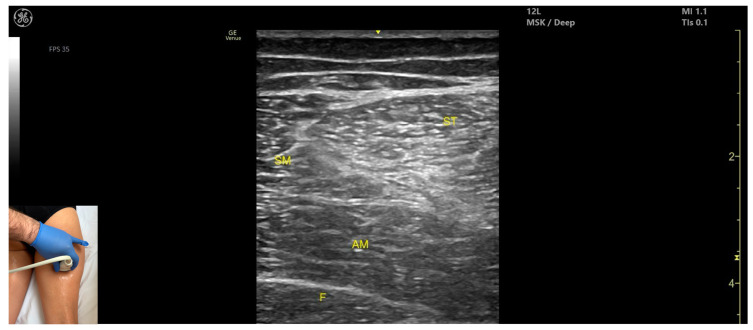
Ultrasound anatomy of *semitendinosus* (ST) with key landmarks: ST—*semitendinosus*; SM—*semimembranosus*; AM—*adductor magnus*; F—femur.
